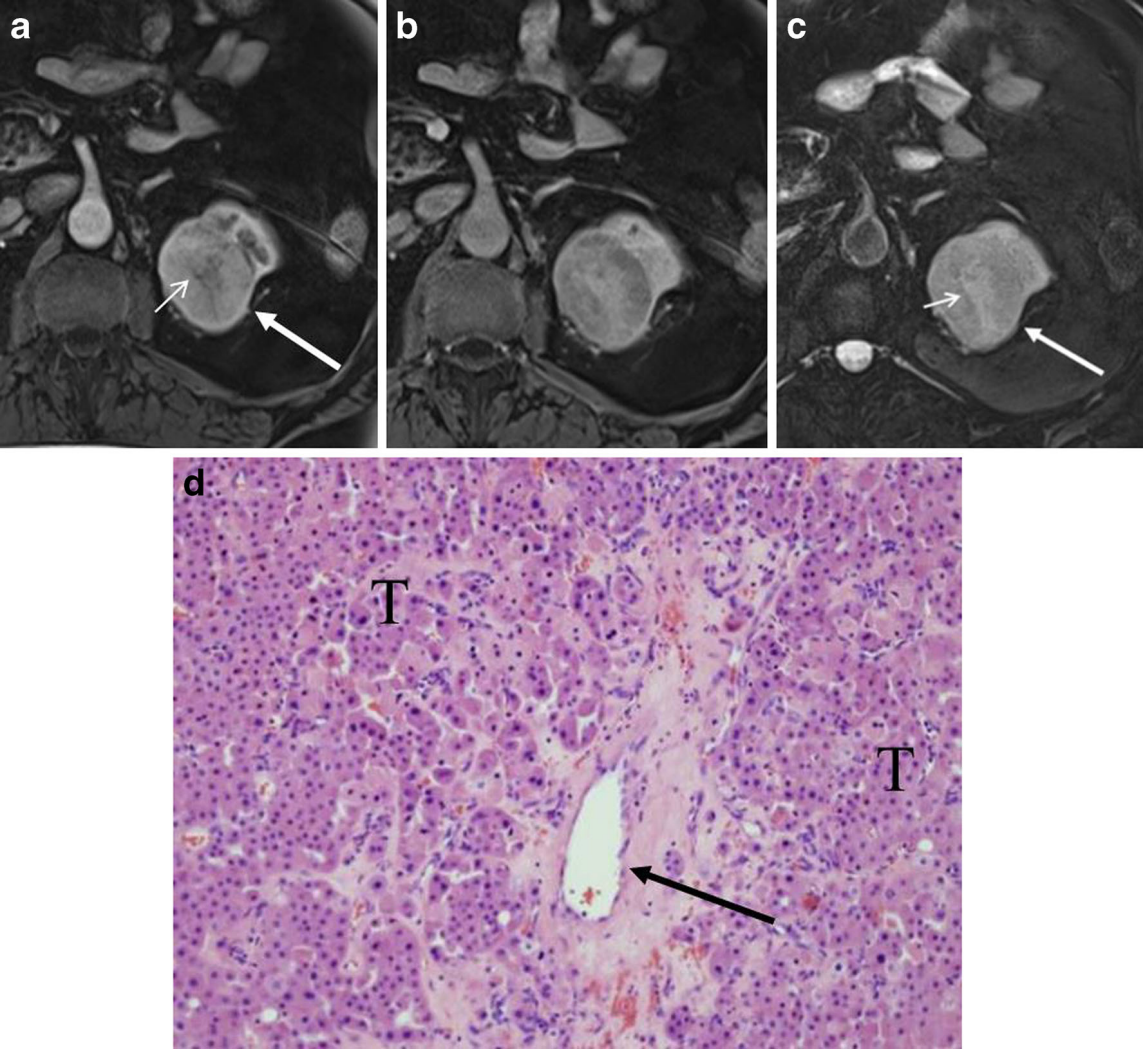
A 71-year-old man with classic renal oncocytoma in the left kidney. a The corticomedullary phase of the axial T1-weighted gradient echo (GRE) magnetic resonance (MR) image with fat saturation demonstrates an arterially enhancing mass (*arrow*) with hypointense central scar (*small arrow*). b The nephrographic phase demonstrates washout with enhancing central scar. c The axial T2-weighted fast spin echo (FSE) image with fat saturation shows the tumour to be of moderate signal (*arrow*) with hyperintense central scar (*small arrow*). d (High magnification) Oncocytoma, organoid pattern, tightly packed nests of tumour cells (*T*) with visible capillaries in the stroma (*arrow*)
